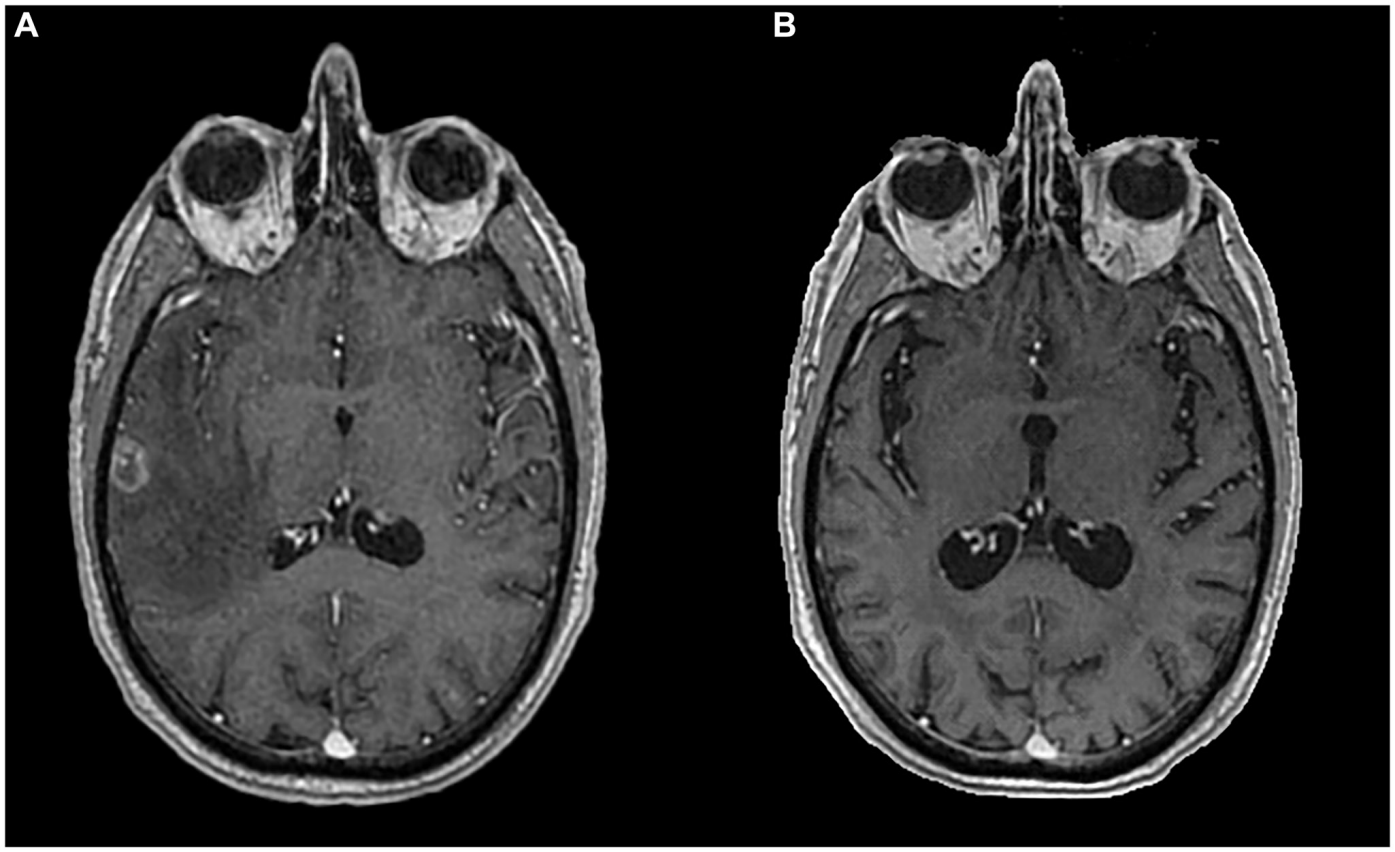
Brain MRI at diagnosis and last Brain MRI performed. The image shows a temporal metastatic lesion at diagnosis (A) and last Brain MRI with maintained complete response (B), in postcontrast 3D T1-weighted.
